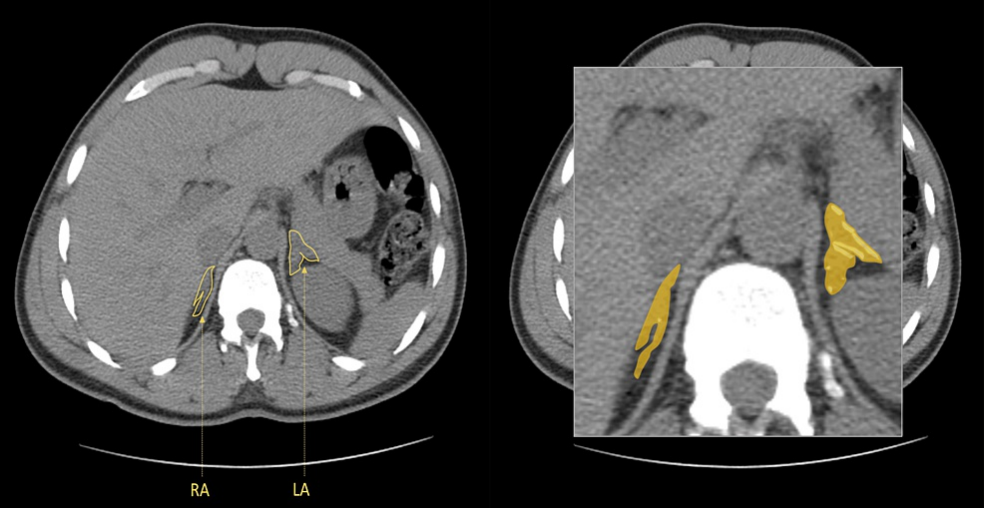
Non-contrast computed tomography scan of the abdomen and pelvis (axial view). Hyperplasia of the adrenal glands (yellow); RA- right adrenal gland; LA - left adrenal gland.
